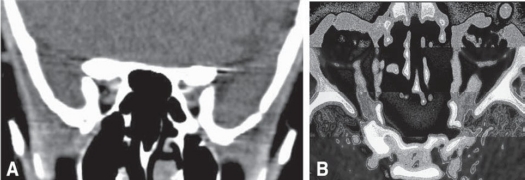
Non-contrast CT images, coronal and axial CT scan showed linear calcification of the nerve suggestive of a tram track appearance with mild hyperostosis.
